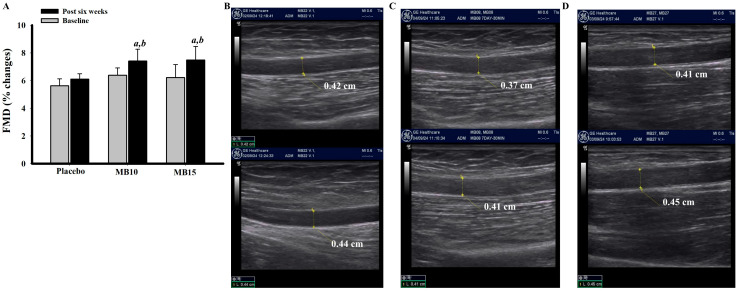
Flow-mediated dilation (FMD) of volunteers before and six weeks after the intervention (A). Representative ultrasonographic image elucidates brachial artery diameter before occlusion (upper panel) and reperfusion (lower panel) of placebo group (B), MB10 (C), and MB15 (D) performed after six weeks of intervention. Data are presented as mean ± SEM of %changes of baseline (n = 9/group). *^a^ p* > 0.05 when compared with baseline, *^b^ p* < 0.05 when compared with placebo (post six weeks).
